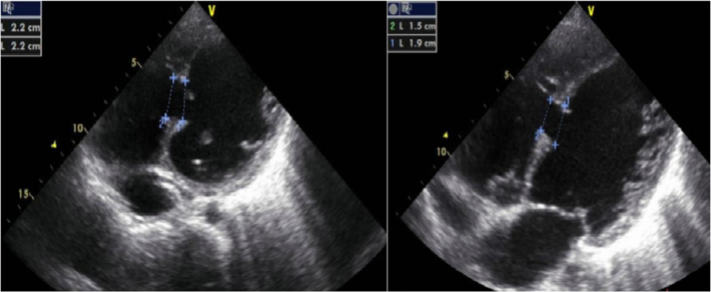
Diameters of VSD on transthoracic echocardiogram.
